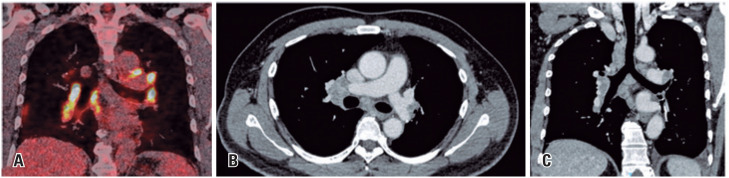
Following the initiation of treatment, the coronal 2-deoxy-2-[fluorine-18] fluoro- D-glucose ^18^F-FDG)-PET/CT view (A) revealed primary tumor and secondary lymph node response but also enlarged lymph nodes with increased FDG uptake (SUVmax 36.9) in the mediastinal chain and pulmonary hila. Subsequent post-operative axial and coronal chest CT images with mediastinum window settings (B and C) demonstrated multiple enlarged mediastinal and hilar lymph nodes with heterogeneous enhancement. In this clinical context, the possibility of sarcoid-like reactions induced by immunotherapy was suspected
